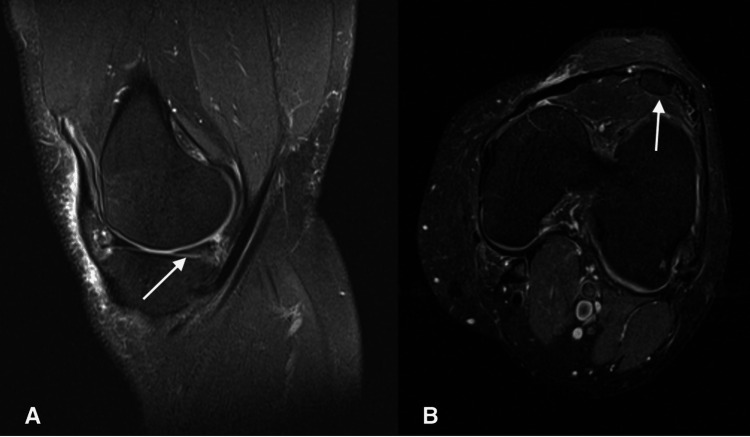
(A) Sagittal view of MRI reveal grade 2 intrasubstance posterior horn meniscus tear (arrow); (B) Axial view of the MRI reveal hypoplastic patella (arrow)
